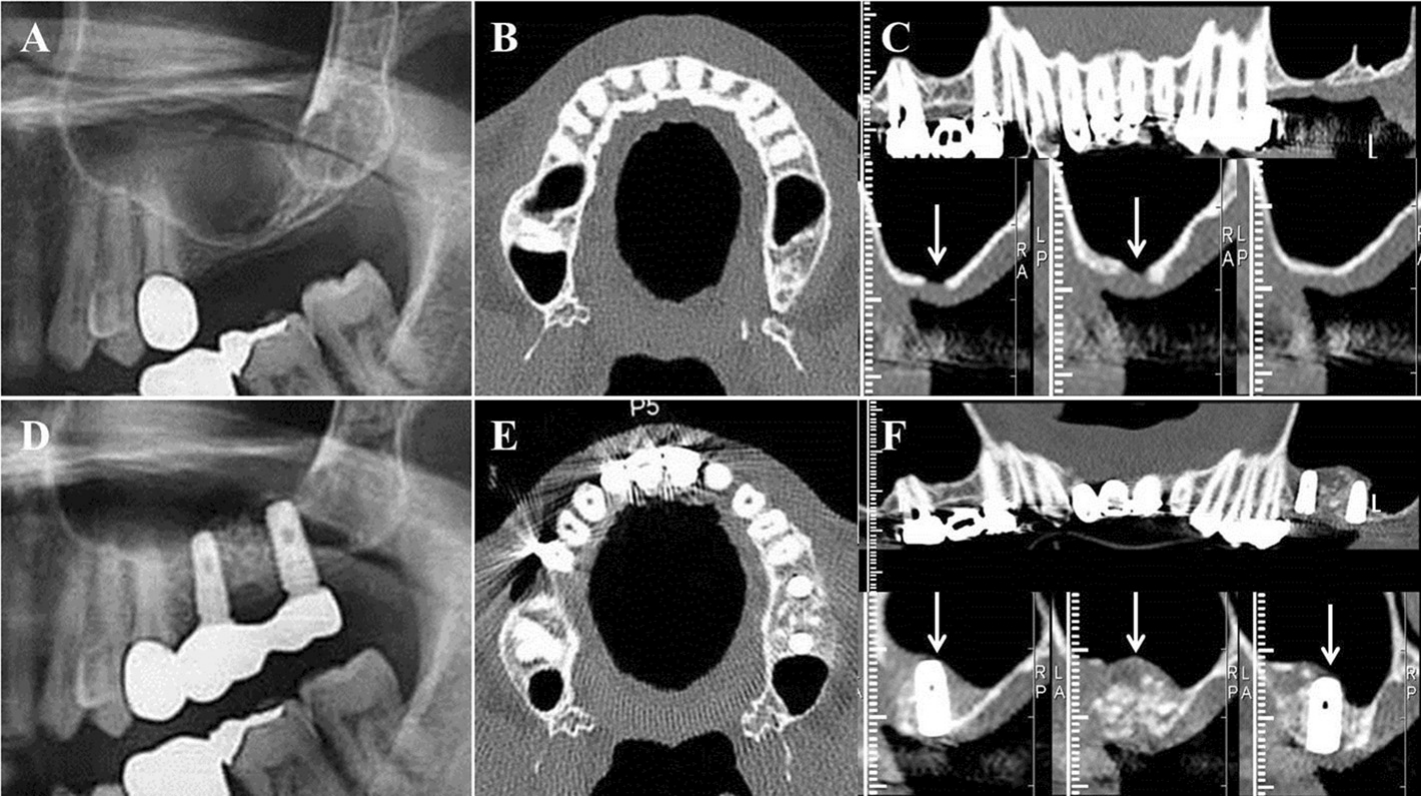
Panoramic and computerized tomographic (CT) evaluation of the subantral inlay jaw bone graft site. a–c Preoperative panoramic and CT views (axial, coronal, and sagittal) show a thin alveolar bone height in left maxillary posterior ridge, even perforation of alveolar bone is observable (arrows). d–f Radiographs at 6 months after surgery (T3) showed the stabilization of implant fixtures with newly generated bone in the maxillary sinus floor (arrows)
